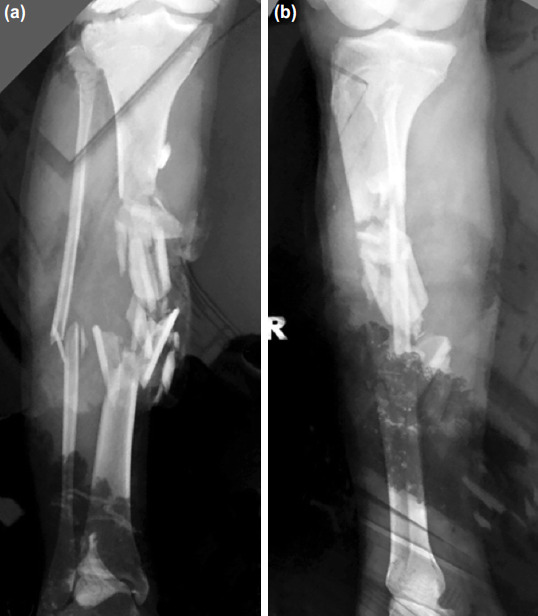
(a) Radiographs with anteroposterior (b) and lateral views of the right tibia showing severe comminuted fracture of the middle third of the tibia.
